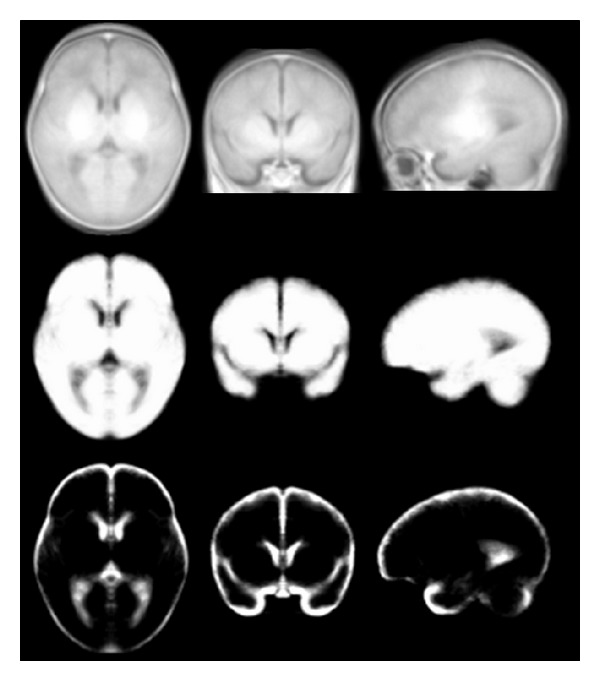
Symmetric template (upper row), probabilistic models of brain (middle row), and CSF (lower row) created from T1-weighted MR images of newborns aged between 39 and 42 weeks.
